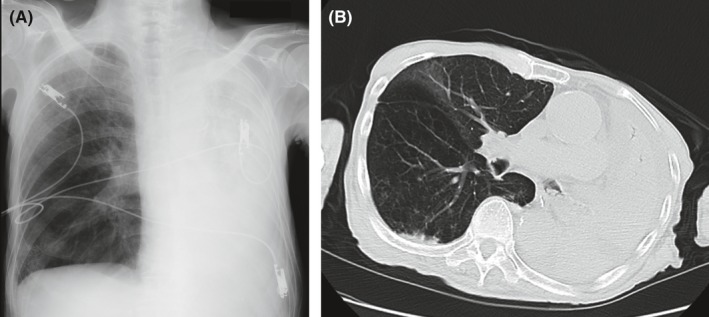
Chest radiograph (A) and CT scan (B) revealing complete atelectasis of the left lung
